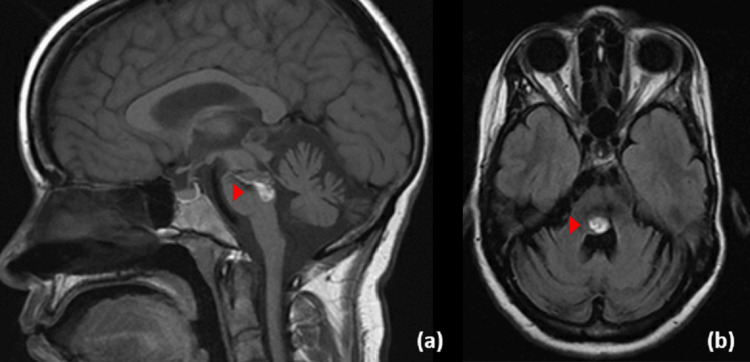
Follow-up contrast magnetic resonance imaging Sagittal (a) and axial (b) cut of magnetic resonance imaging showing a contrast-enhancing lesion with a newly appearing focal area of subacute bleeding noted at the site of the known Ponto-mesencephalic cavernoma with associated old blood degradation products.
